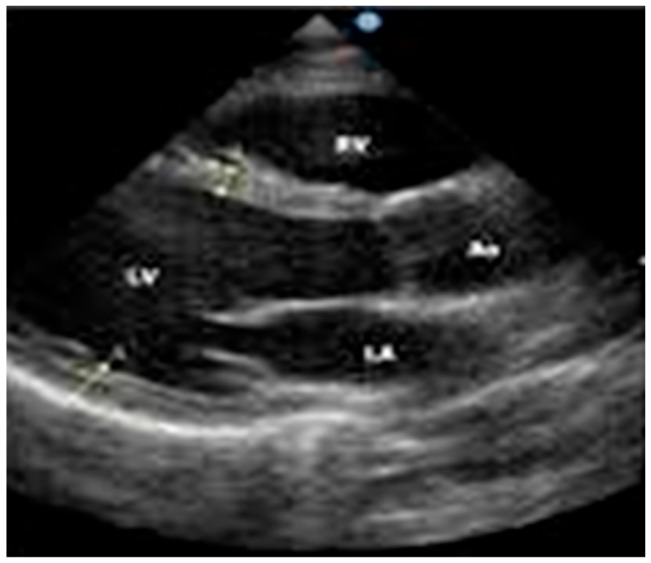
Cardiac transesophageal ultrasound—Foramen ovale slope with intermittent, right-to-left blood shunting.
